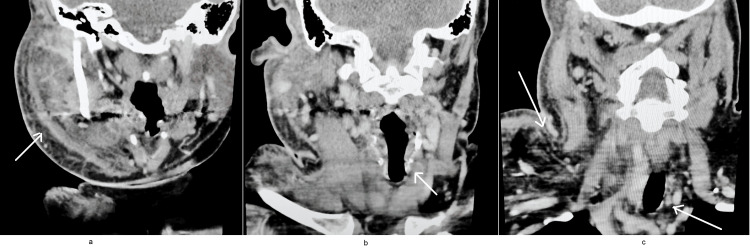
CT Neck a: Diffuse soft tissue swelling and inflammation of the right side of the face b: Swelling and inflammation of the right side of the neck with resulting tracheal deviation to the left c: Soft tissue swelling and inflammatory changes extending down to the upper chest, with distal tracheal deviation to the left.
